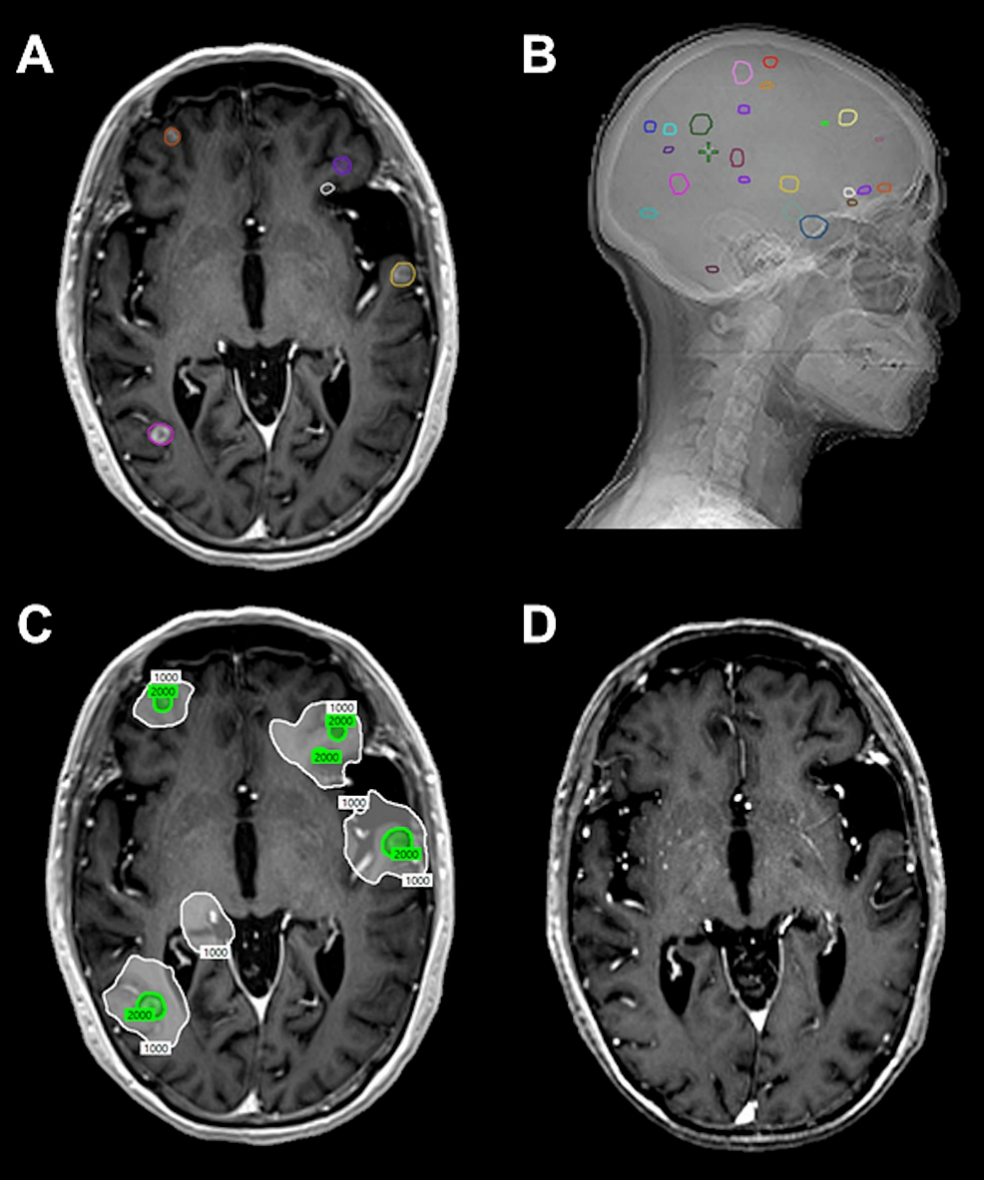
Treatment Planning and Follow-Up Imaging (A) Representative axial slice from treatment planning magnetic resonance imaging showing five treated gross tumor volumes. (B) Representative lateral digitally reconstructed radiograph showing the distribution of 23 treated lesions. (C) Representative axial slice showing radiation treatment plan isodose lines including the prescription dose (20 Gy, green) and the 50% isodose line (10 Gy, white). (D) Representative axial slice from follow-up magnetic resonance imaging showing treatment response and disease surveillance.
